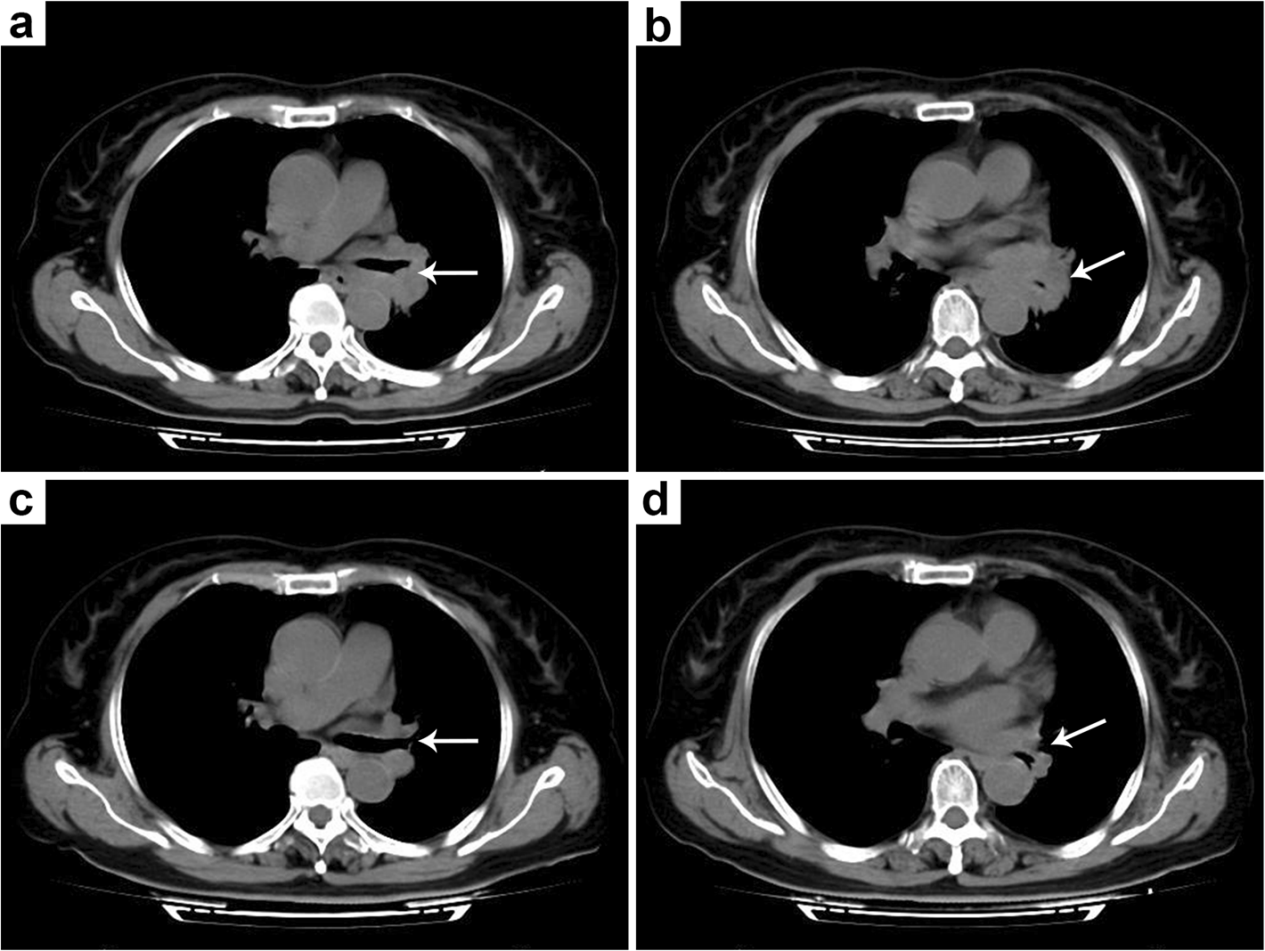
Four computed tomography (CT) images of the chest before and after treatment. a The left upper and lower lobar bronchi at the entrance are obstructed (arrow). b A mass in the left lung hilar region (arrow), which is indistinguishable from the surrounding tissue. c The original obstructed bronchi are unobstructed after treatment (arrow). d The original mass disappeared after treatment (arrow), and the thoracic aorta and pulmonary vessels are clearly defined
